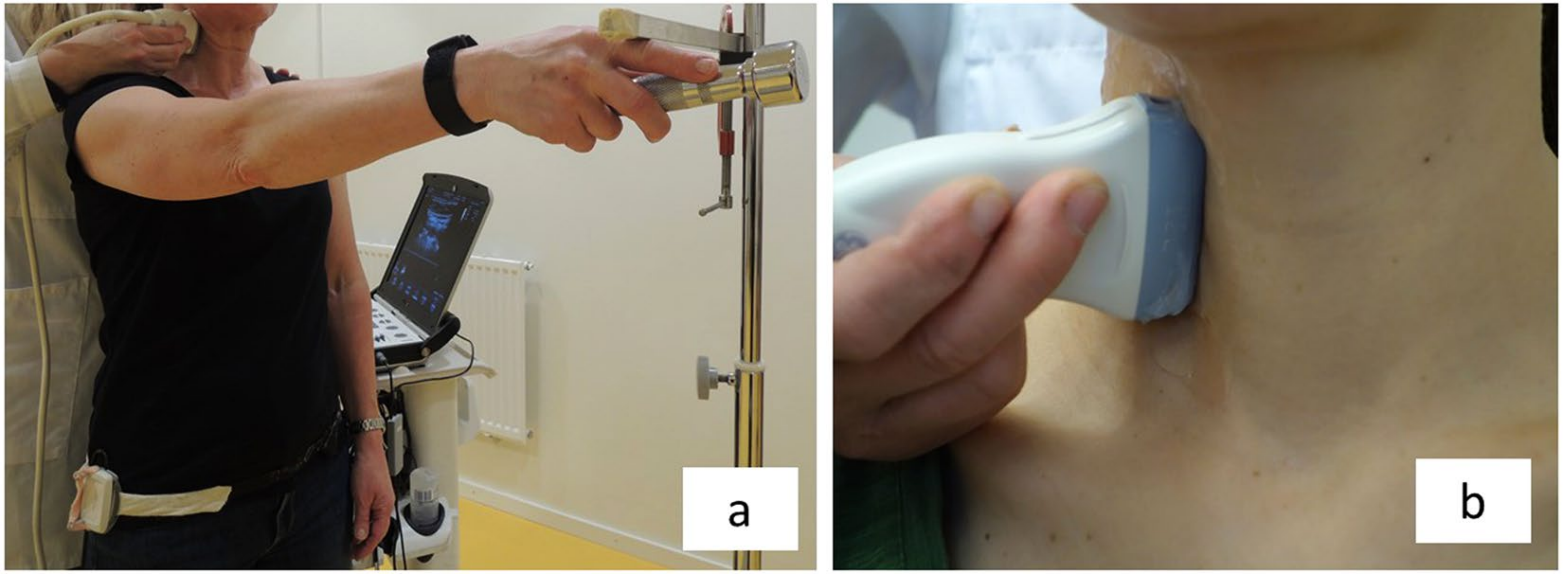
Ultrasound measurement. (a) Ultrasound imaging of ventral neck muscles during arm elevation. The individual held a 0.5 kg (women) or 1 kg (men) weight in the right hand and raised their arm to 90 degrees. An adjustable horizontal bar was fixed with the index finger touching the bar and customized contact switches attached to the individual’s hip and wrist. The contact switches allowed synchronization between the ultrasonography data and arm elevation. The segmental level was verified with a transverse ultrasound projection of the bifurcation of the carotid artery, commonly observed at the C4 level. (b) The transducer was then rotated 90° and orientated longitudinally to the ventral neck muscles.
